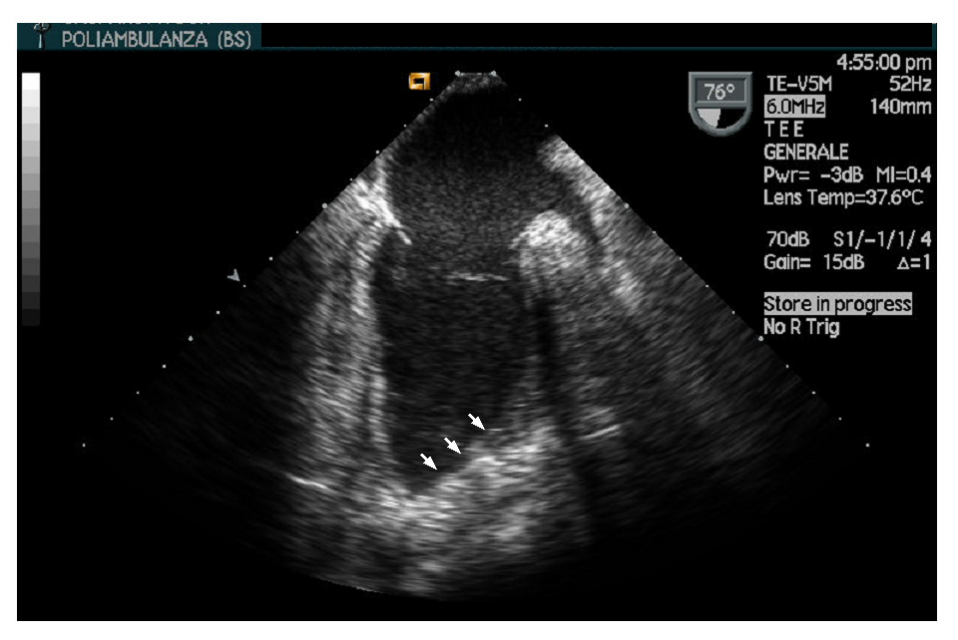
Postoperative transesophageal echocardiographic long axis view of a restored left ventricle. A conical left ventricle is restored. Arrows show the position of the patch. The new apex is evident.
